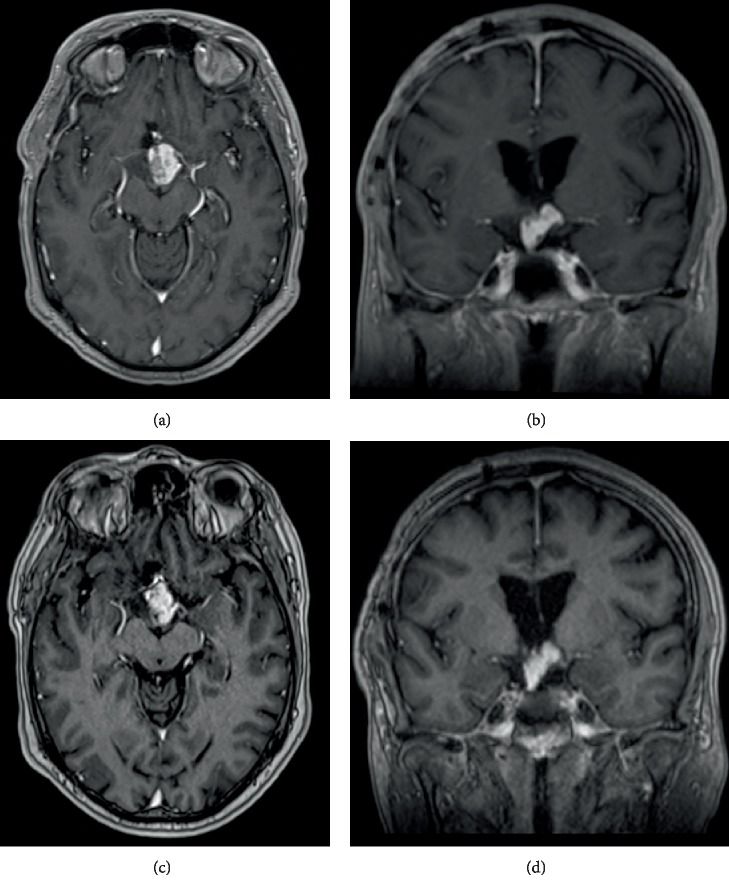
Gadolinium contrast-enhanced MRI of the brain. (a, b) First MRI after re-resection through transcallosal approach shows only partial removal of the tumour was obtained. (c, d) Follow-up study 5 years later shows no progression.
